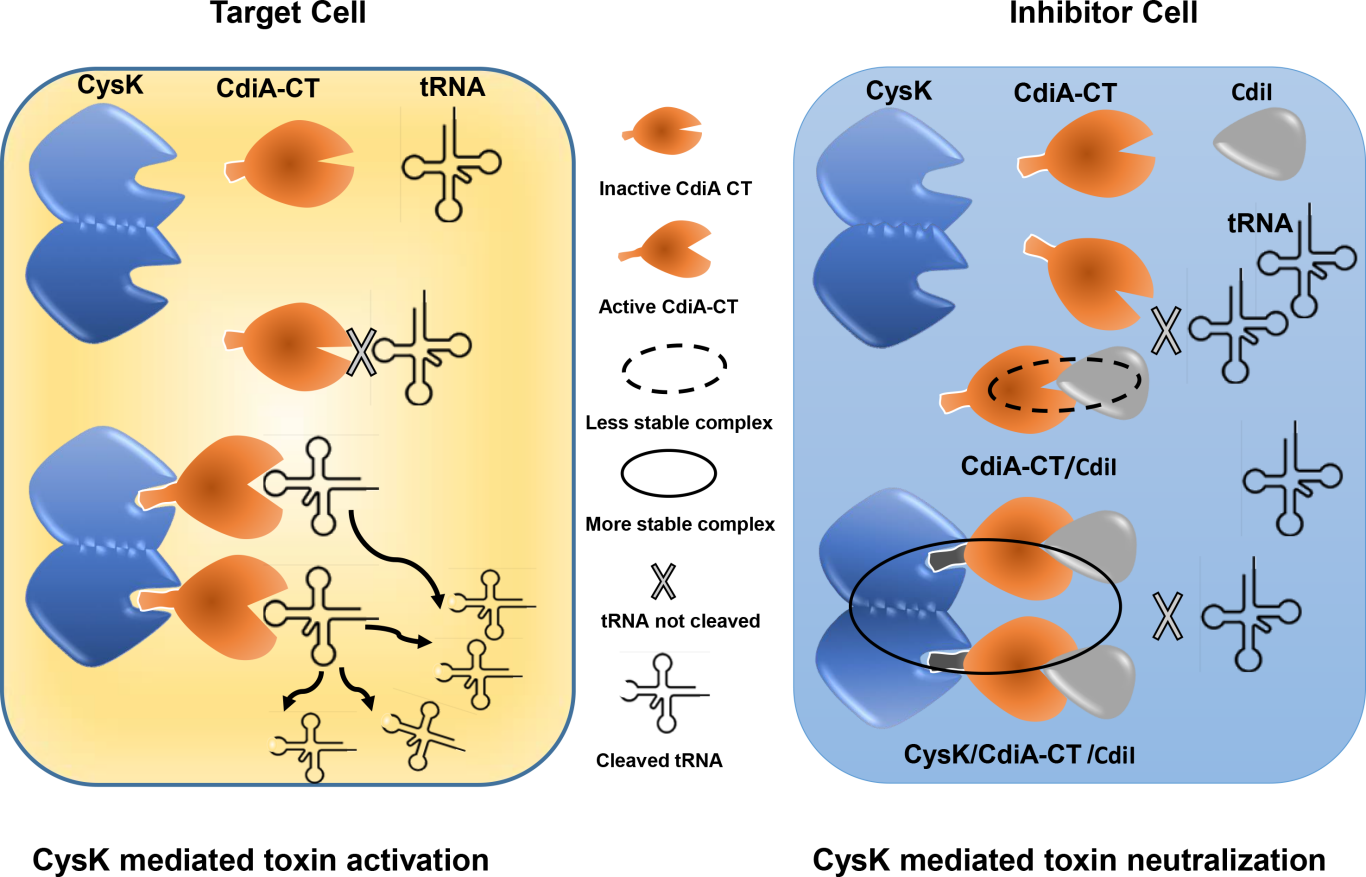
Proposed model for the dual role of CysK in UPEC536 mediated CDI. The left panel shows CysK mediated toxin activation in target cell where CysK dimer binds CdiA-CT and activates tRNAase activity causing growth arrest [23]. The right panel shows the moonlighting role of CysK as a modulator of CdiA-CT ^(M)^/CdiI in the CDI^+^ inhibitory cells, as proposed in the present study. Binding of CysK to CdiA-CT stabilizes CdiA-CT/CdiI immunity complex, primarily by decreasing dissociation rate, to prevent autoinhibition in UPEC536 inhibitor cells.
